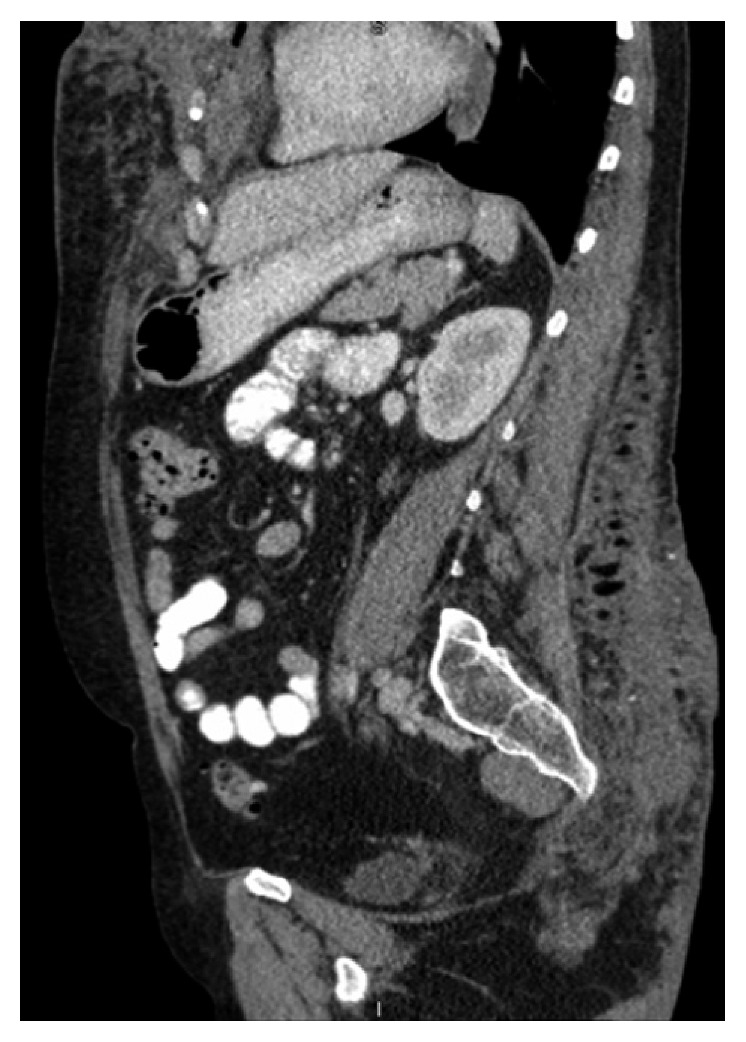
CT abdomen/pelvis 2007: extensive subcutaneous fibrosis involving buttocks.
